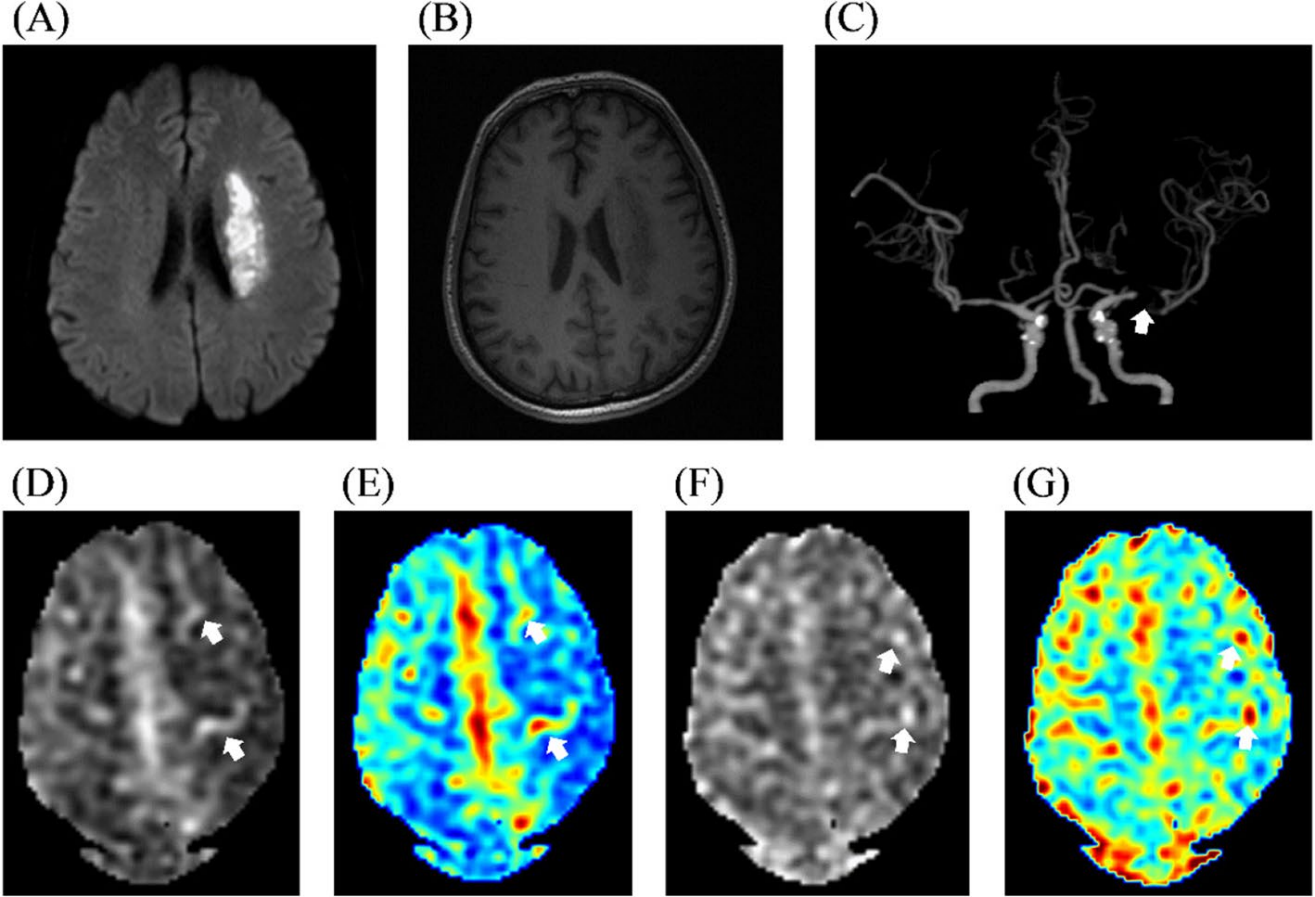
Imaging features of arterial transit artifact. A DWI showed infarction of the left basal ganglia and corona radiata. B Infarction was depicted on 3D-T1WI. C CTA showed occluded MCA and formation of collateral circulation distal to the occlusion. D, E ASL of 1.5 s PLD showed decreased CBF in the left MCA territory and visible arterial transit artifact. F&G, ASL of 2.5 s PLD showed decreased CBF in the left MCA territory and visible arterial transit artifact. DWI: diffusion-weighted imaging; 3D-T1WI: 3-dimensional T1-weighted images; CTA, CT angiography; MCA, left middle cerebral artery; ASL, arterial spin labeling; PLD, postlabeling delay; CBF, cerebral blood flow
